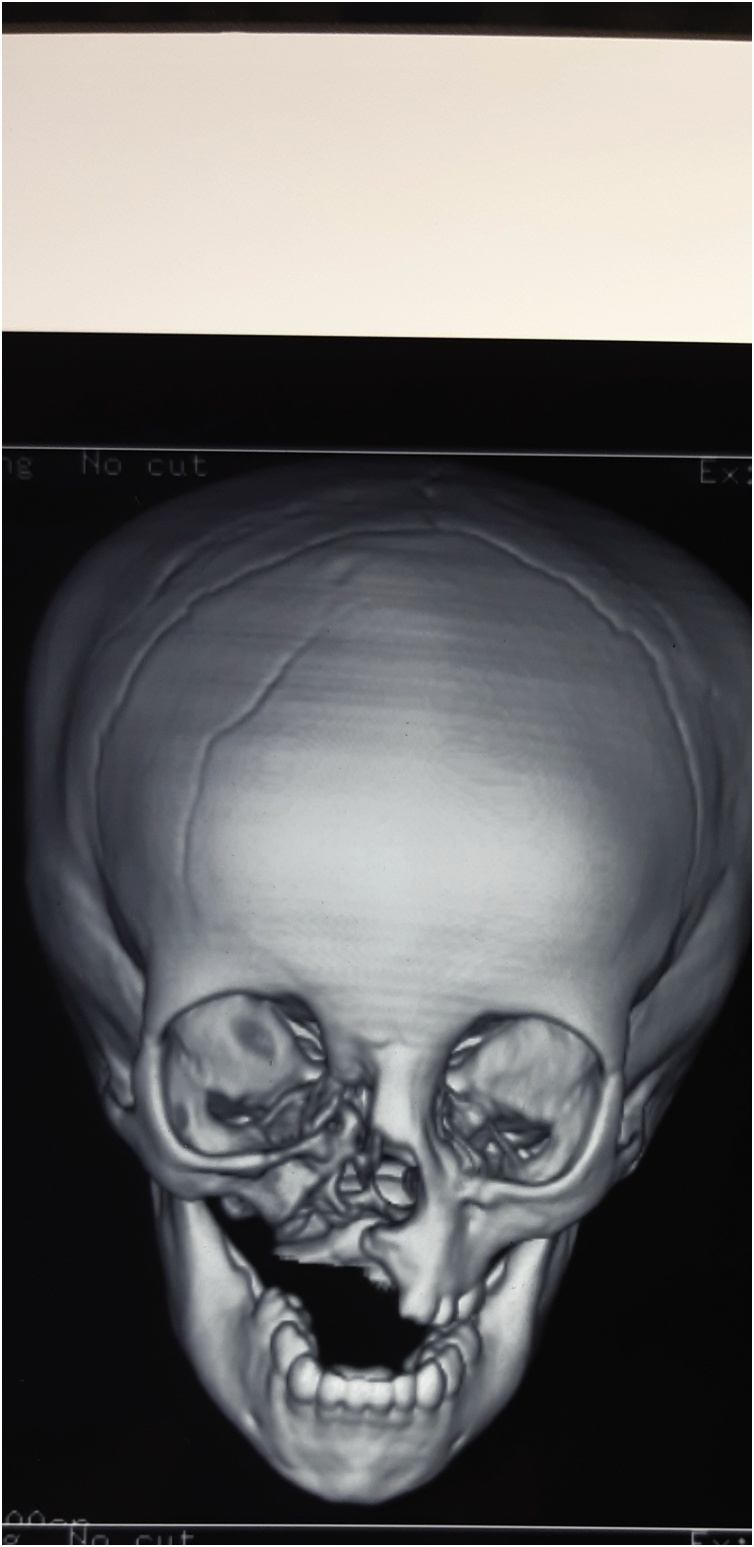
Three-year postoperative 3D reconstruction CT of Patient 1.
